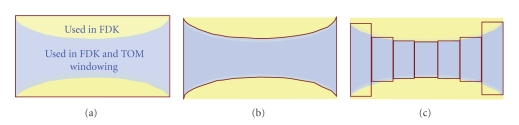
The detector utilization of the FDK-based and TOM windowing reconstruction approaches. The area closed by red lines represents the actual acquired area of the detector for (a) multi-axial scan/line source CT, (b) line source CT curved collimator, and (c) multi-source inverse geometry CT. The area in blue represents the data required to reconstruct volume using TOM windowing approach. Note that TOM windowing has an advantage over FDK in detector utilization if data is acquired by the line-source CT with the collimator or the multi-source inverse geometry CT.
